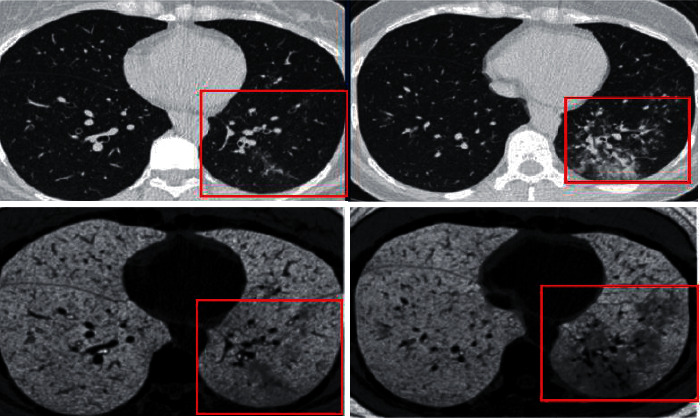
Lung CT images of COVID-19 findings before and after the process. The findings are in (red frame).
